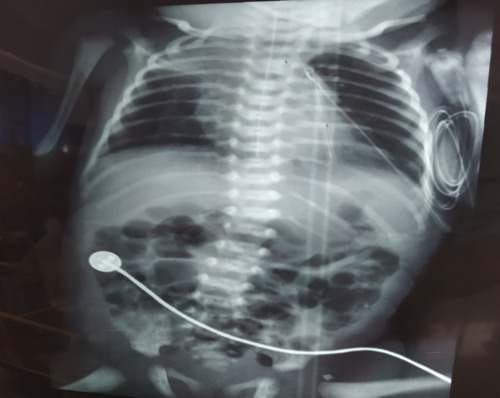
Third post-operative day chest radiograph showing adequate lung expansion and a right upper zone patch.
